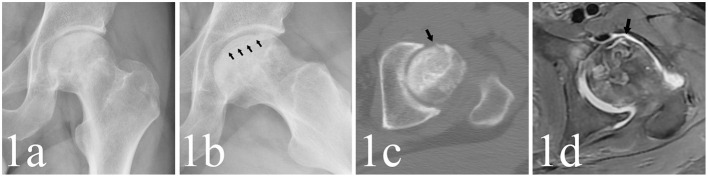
(a,b) A 29-year-old male patient whose left hip appeared as crescent sign and collapsed in FL view (as the black arrows point) but not in AP view. (c,d) The CT and MRI detected subchondral fracture in anterior femoral head (as the black arrows point).
